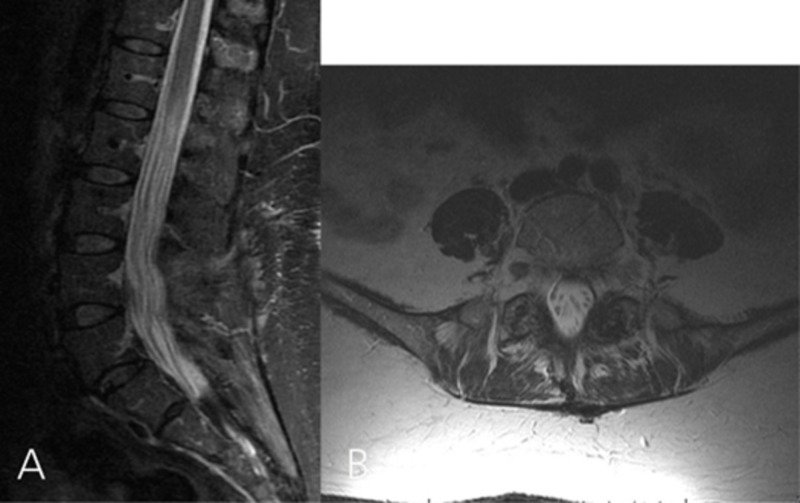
Repeated lumbar MRI done 11 months after the surgery. A: Sagittal T2-weighted image showing no residual canal stenosis. B: Axial T2-weighted image showing that the cystic lesion involving the left L5-S1 facet complex has resolved completely
